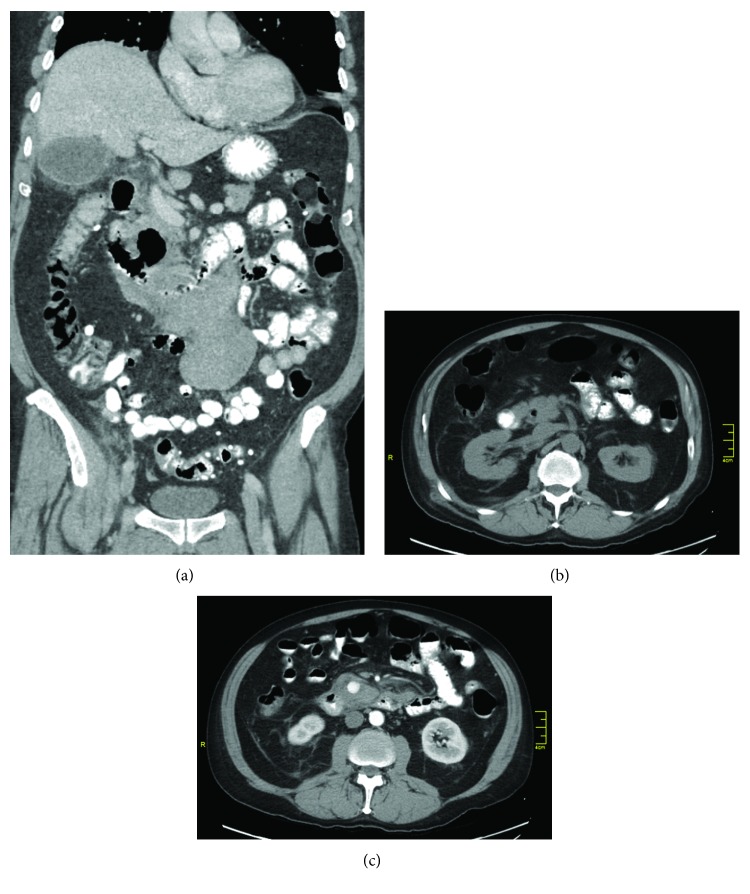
(a–c) CT-scan: (a) large duodenal diverticulum (arrow), mesenteric hematoma, stranding of surrounding tissue. (b) Contrast in diverticulum (arrow). (c) Two cm pseudoaneurysm with hematoma (arrow).
